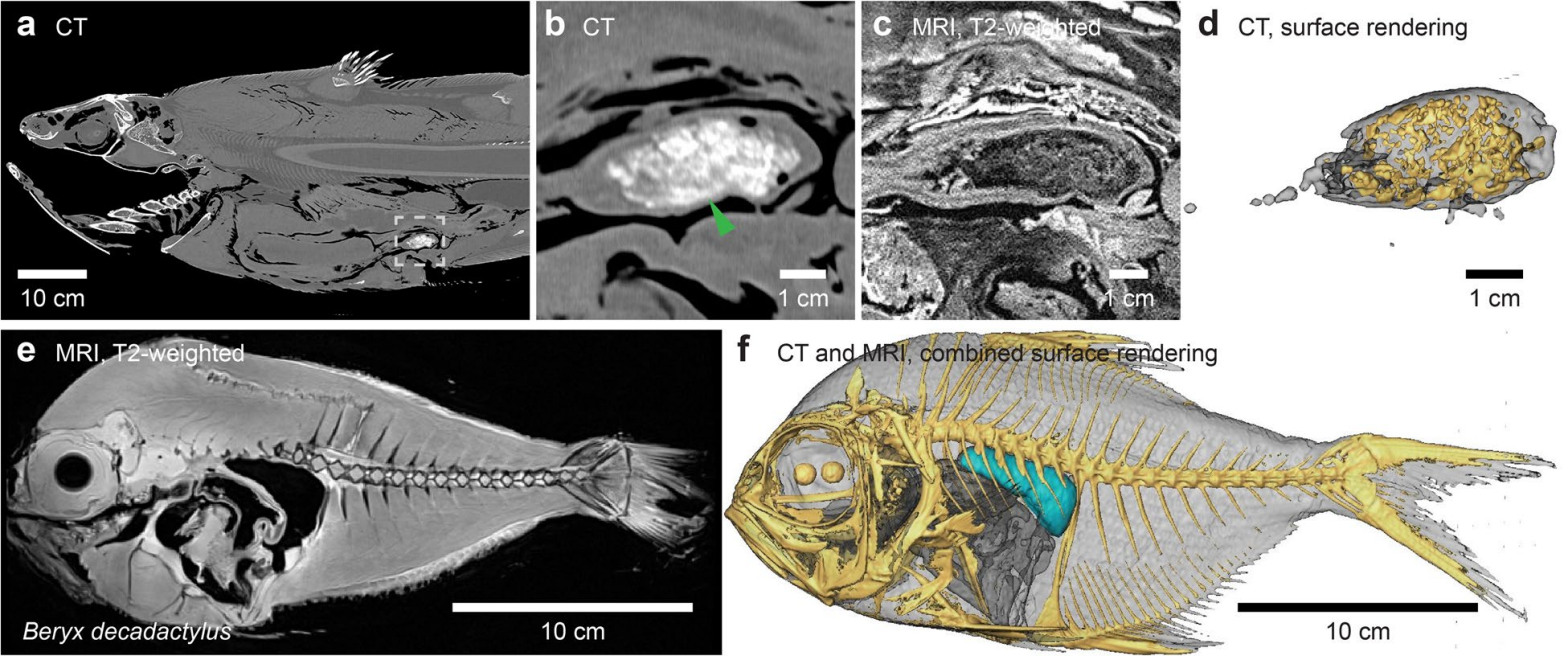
Prey items in gastrointestinal tract and prey with a swim bladder. a–d Mineralized remains of prey items in the distal portion of the digestive tract of the coelacanth imaged by X-ray computed tomography (CT) and magnetic resonance imaging (MRI). Dashed gray square in a is magnified in b and shown in a similar view plane using T2-weighted MRI (c). A three-dimensional surface rendering of the fecal matter is shown in d. e,f Sagittal T2-weighted MRI slice (e) and three component (skin, bones, swim bladder) model (f) made from CT and MRI of *Beryx decadactylus*, a known prey item of the coelacanth. This species contains an air-filled swim bladder (light blue segment in f) that under the assumption of neutral buoyancy displaces a volume of seawater with the same mass as the net weight of the fish (excluding the swim bladder) in seawater
